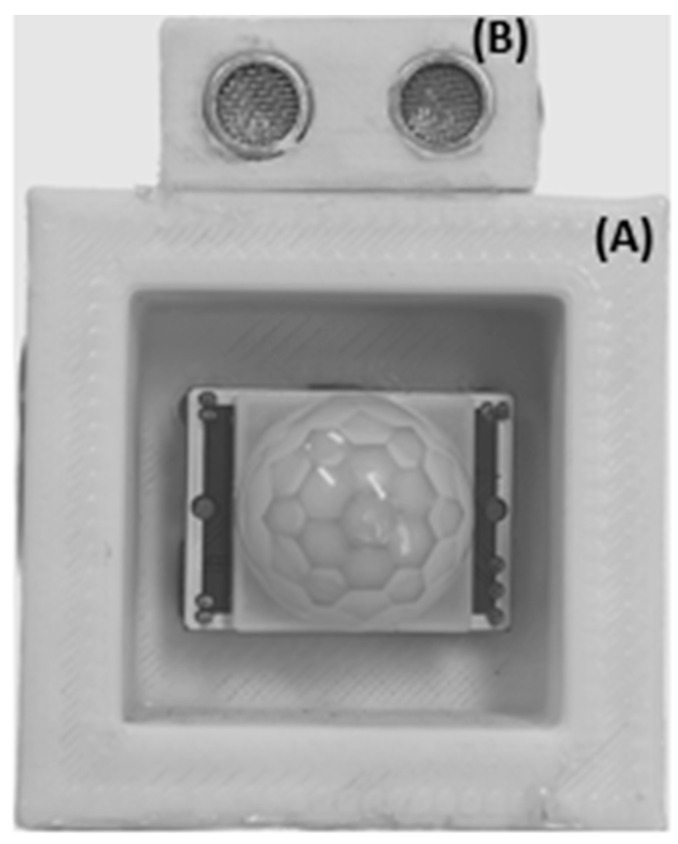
(A) is the box built for each PIR sensor to reduce its FOV. (B) is the box built for each ultrasonic sensor installed on top of the related PIR sensor.
